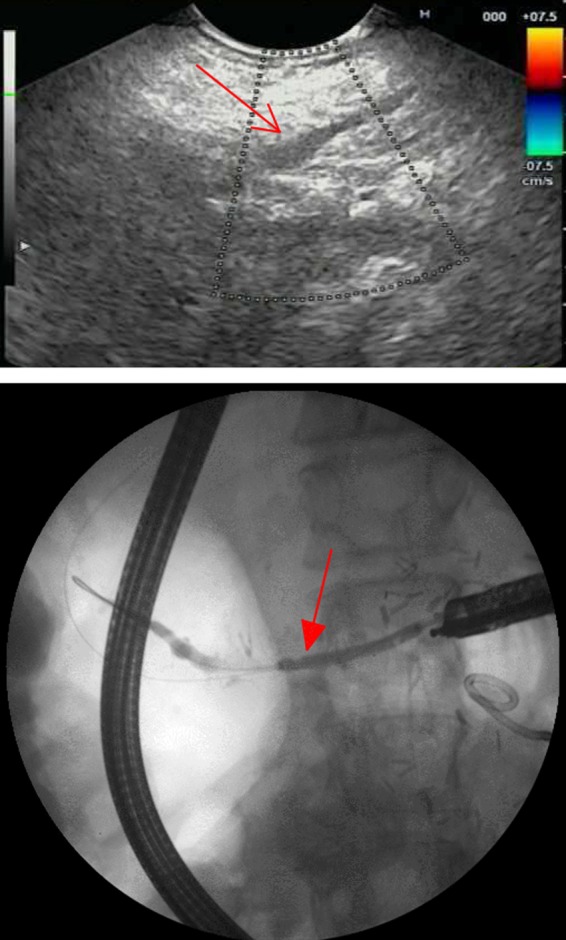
Top—EUS image highlighting a dilated pancreatic duct (simple arrow). Bottom—Fluoroscopy image demonstrating adequate placement of a 7F 9-cm Hobbs stent with internal flange across the newly dilated pancreaticojejunostomy (filled-head arrow). EUS, endoscopic ultrasound.
